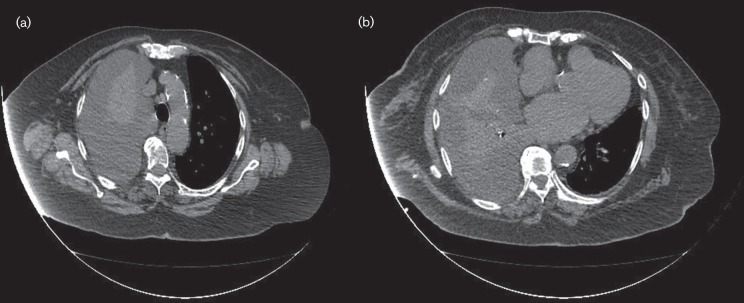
Patient’s CT scan showing the presence of calcified atherosclerotic plaques of the aortic arch (a) and descending aorta (b).
